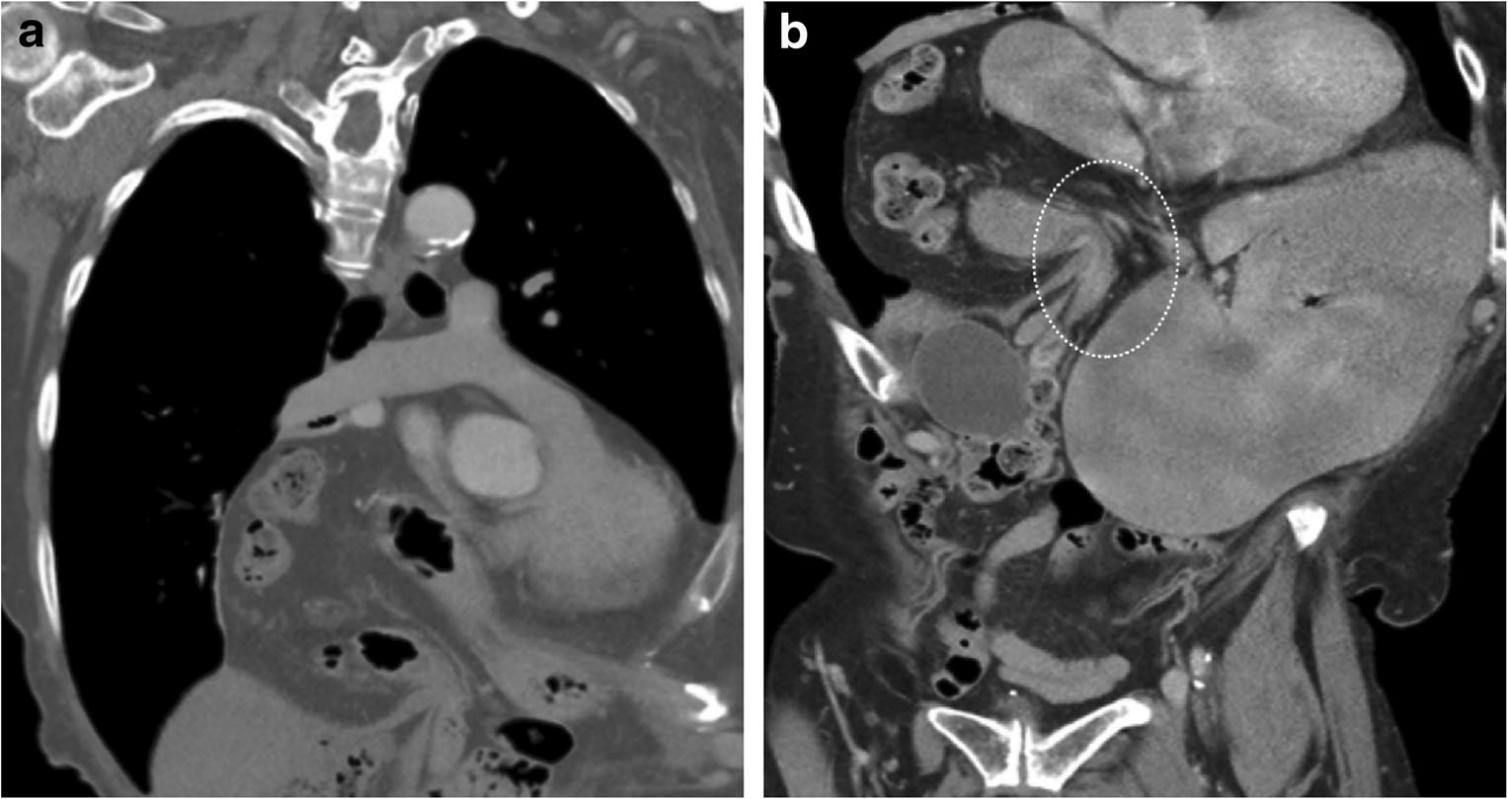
a, b An 86-year-old male with history of (h/o) melanoma, now presenting with chronic right upper abdominal pain. Coronal contrast CT (a) identifies a right retrosternal diaphragmatic hernia. Note the narrow neck of the hernia sac with herniation of the stomach (body, pylorus and antrum), colon and omentum into the mediastinum. This is an incarcerated hernia. The patient was offered elective repair, which was declined. The same patient presented with new onset of acute right upper quadrant pain after 2 months. Coronal contrast-enhanced CT (b) demonstrates severe dilation of the stomach with gastric outlet obstruction (Movie 4). The herniated hepatic flexure is not dilated. Adhesions within the hernia sac can lead to bowel obstruction and narrowing. These require urgent decompression of the distended stomach by placement of a gastric tube followed by surgical repair
